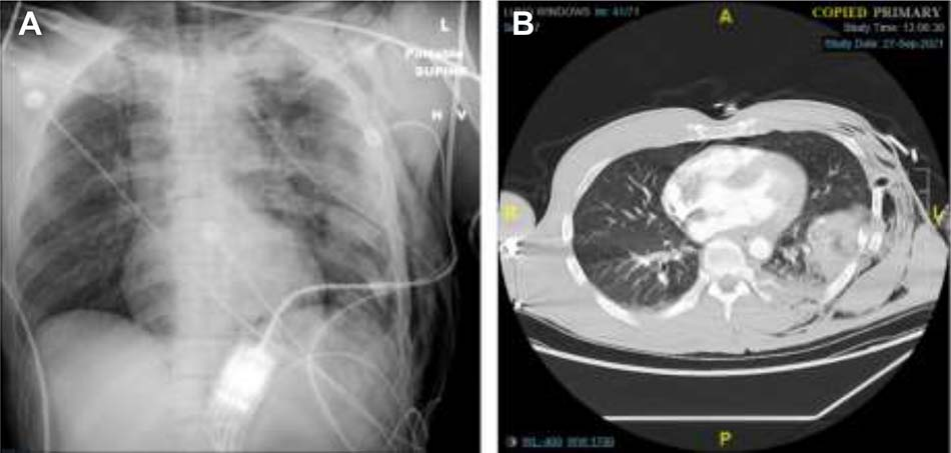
Chest X-Ray and CT imaging showing rib fracture. (A) Chest X-ray and (B) CT showing fractures to the left ribs.
